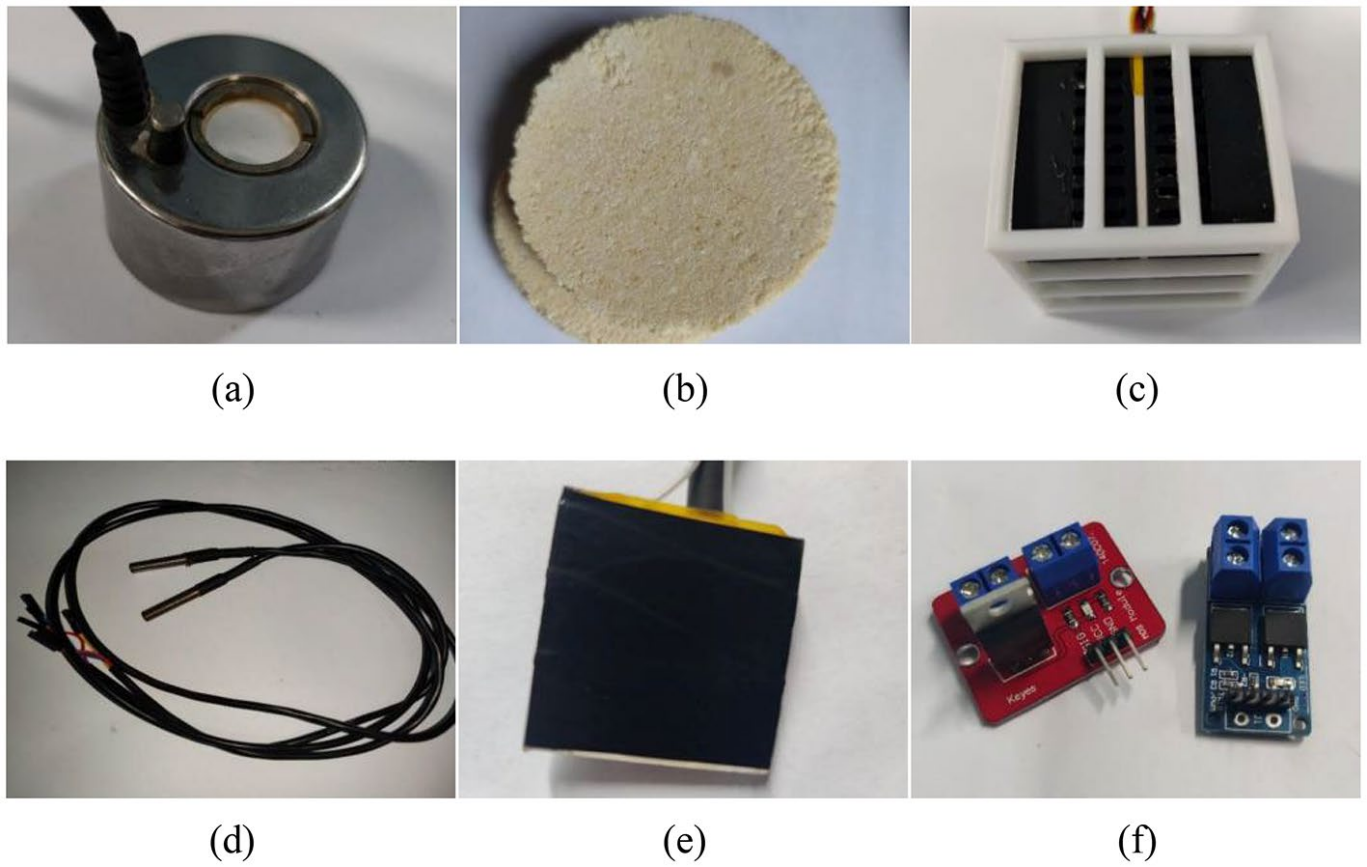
The experimental apparatus. (a) Ultrasonic atomizer. (b) Smoke cartridges. (c) Heating device. (d) Temperature sensor. (e) Target. (f) MOS transistor.
